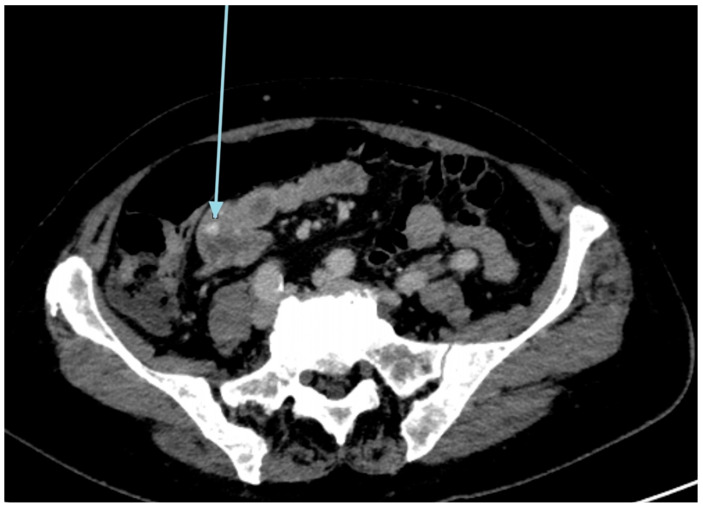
Angio CT contrast blushing in the proximal small bowel (late image).
